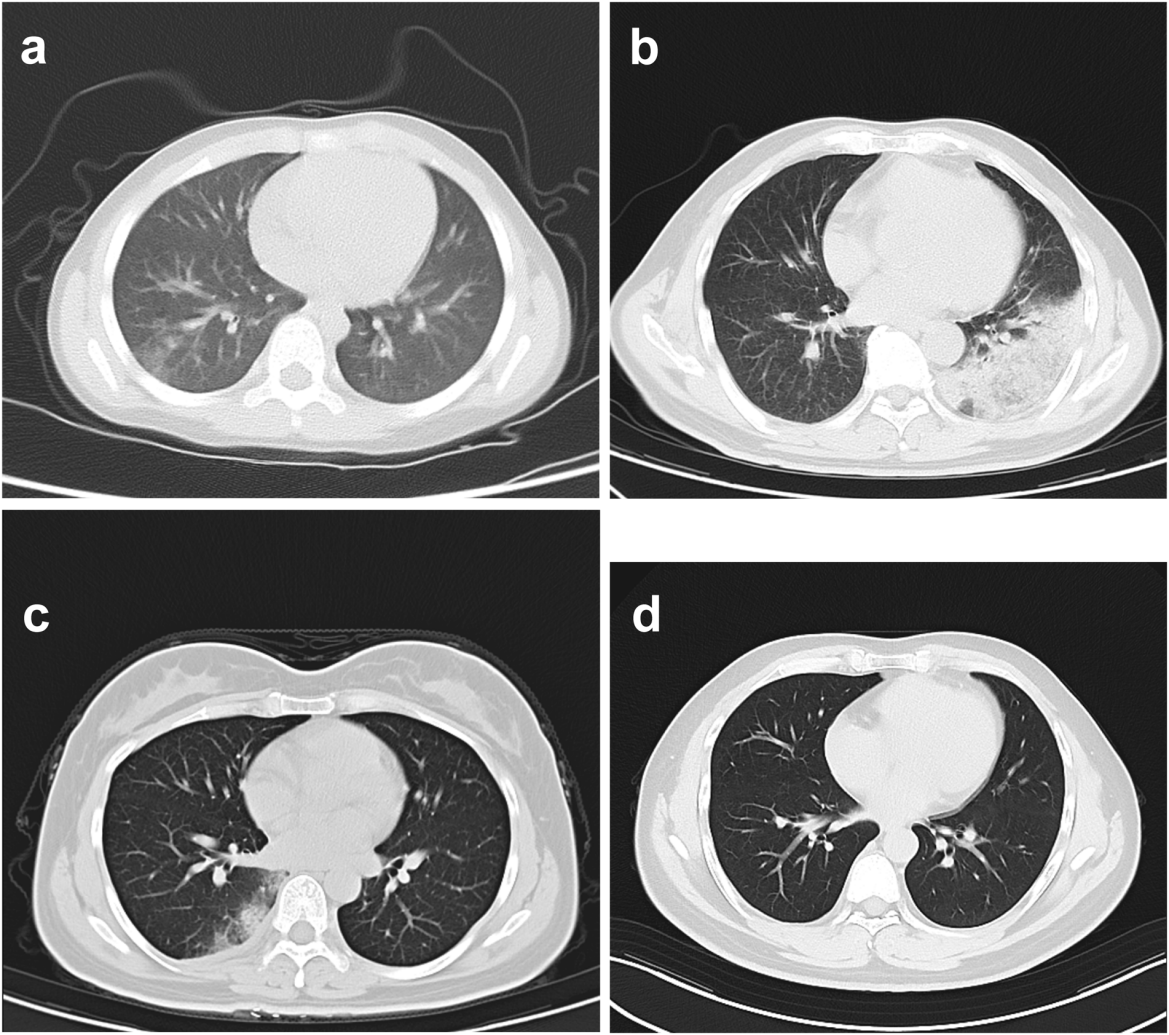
Chest CT indicates inflammation and other lesions in the lung of four patients. (A) Chest CT in case 1; (B) Chest CT in case 2; (C) Chest CT in case 3; (D) Chest CT in case 4.
